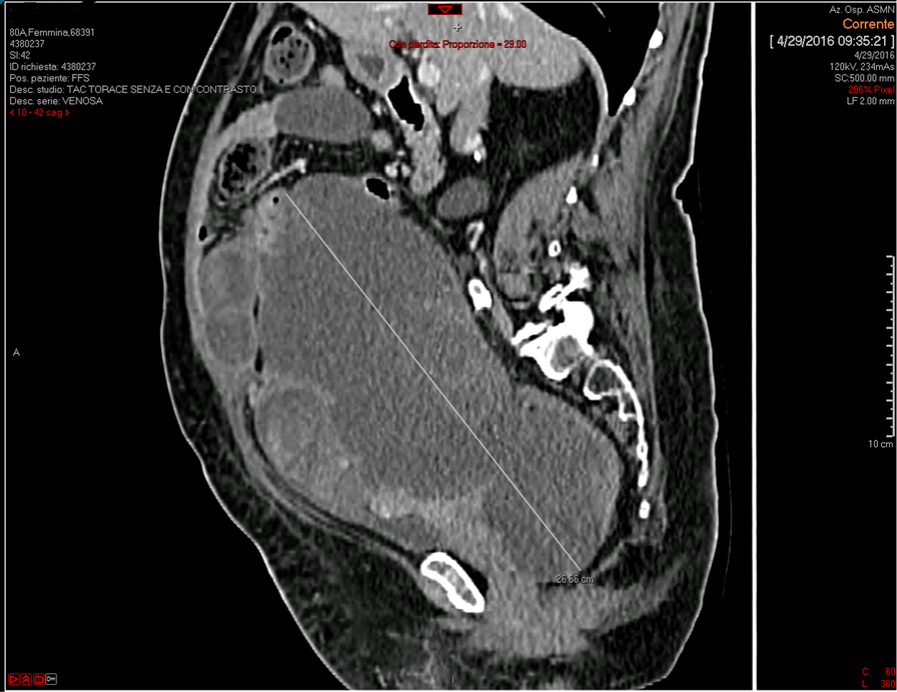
Pre-chemotherapy computed tomography scan taken 4 weeks after surgery revealing a new, multicystic mass (27 × 15 cm)
